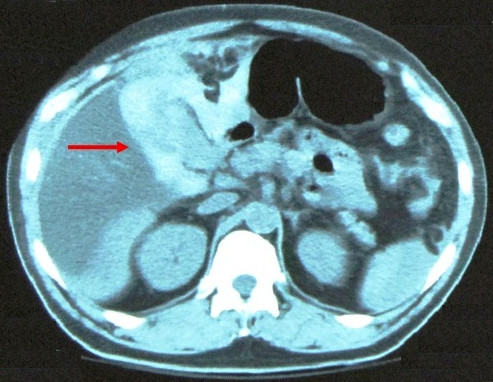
Computed tomography (CT) scan reveals pericholecystic fluid (arrow) and indicates the potential for some kind of injury of the gallbladder.
